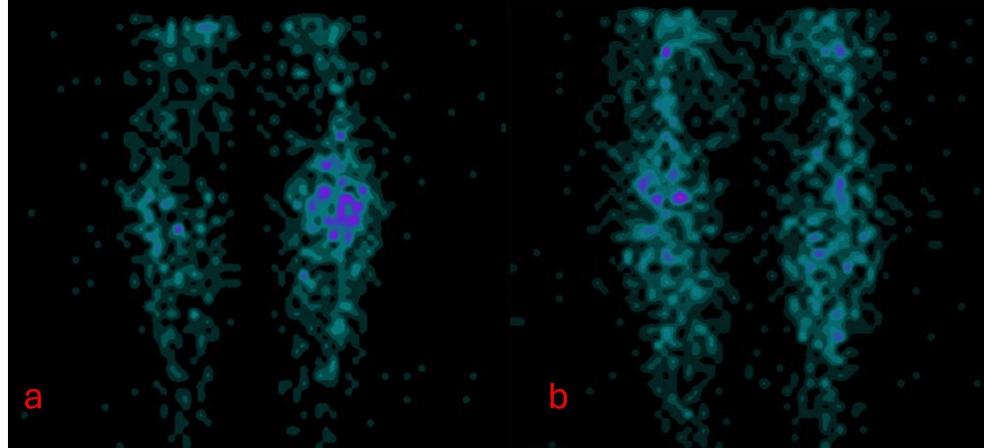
Bone scintigraphy, flow phase with 99mTc-MDP (a) Anterior view of the flow phase with 99mTc-MDP through the lower limbs. (b) Posterior view of flow phase with 99mTc-MDP through the lower limbs.
